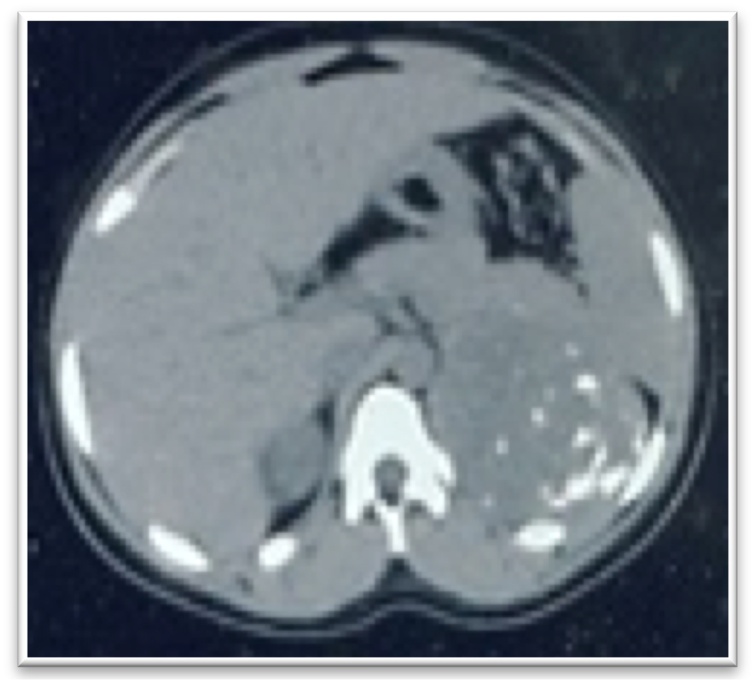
Abdominal CT showing left adrenal mass with calcifications (case 3).
